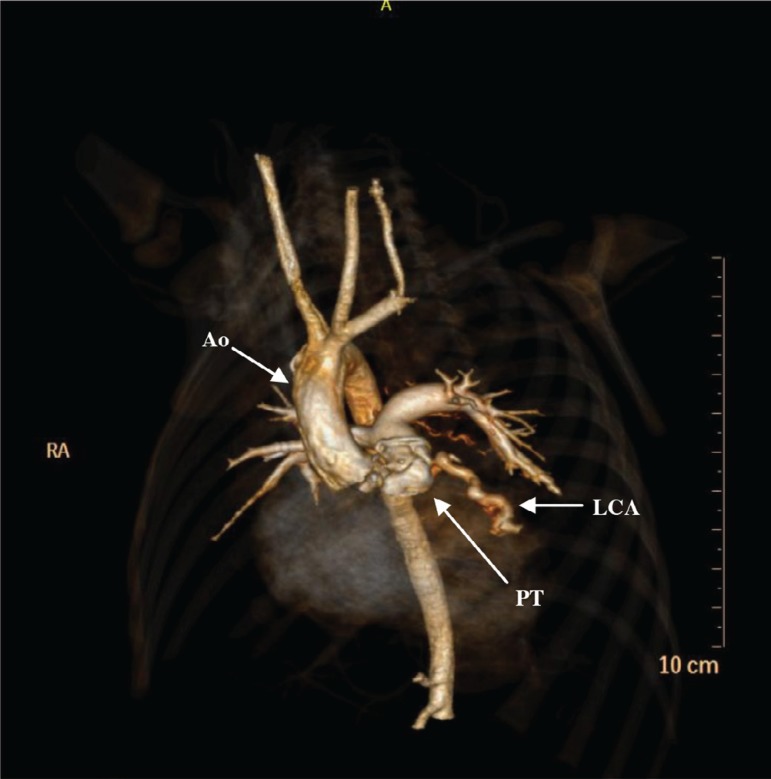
A 3D volume-rendered computed tomographic angiography showing anomalous
